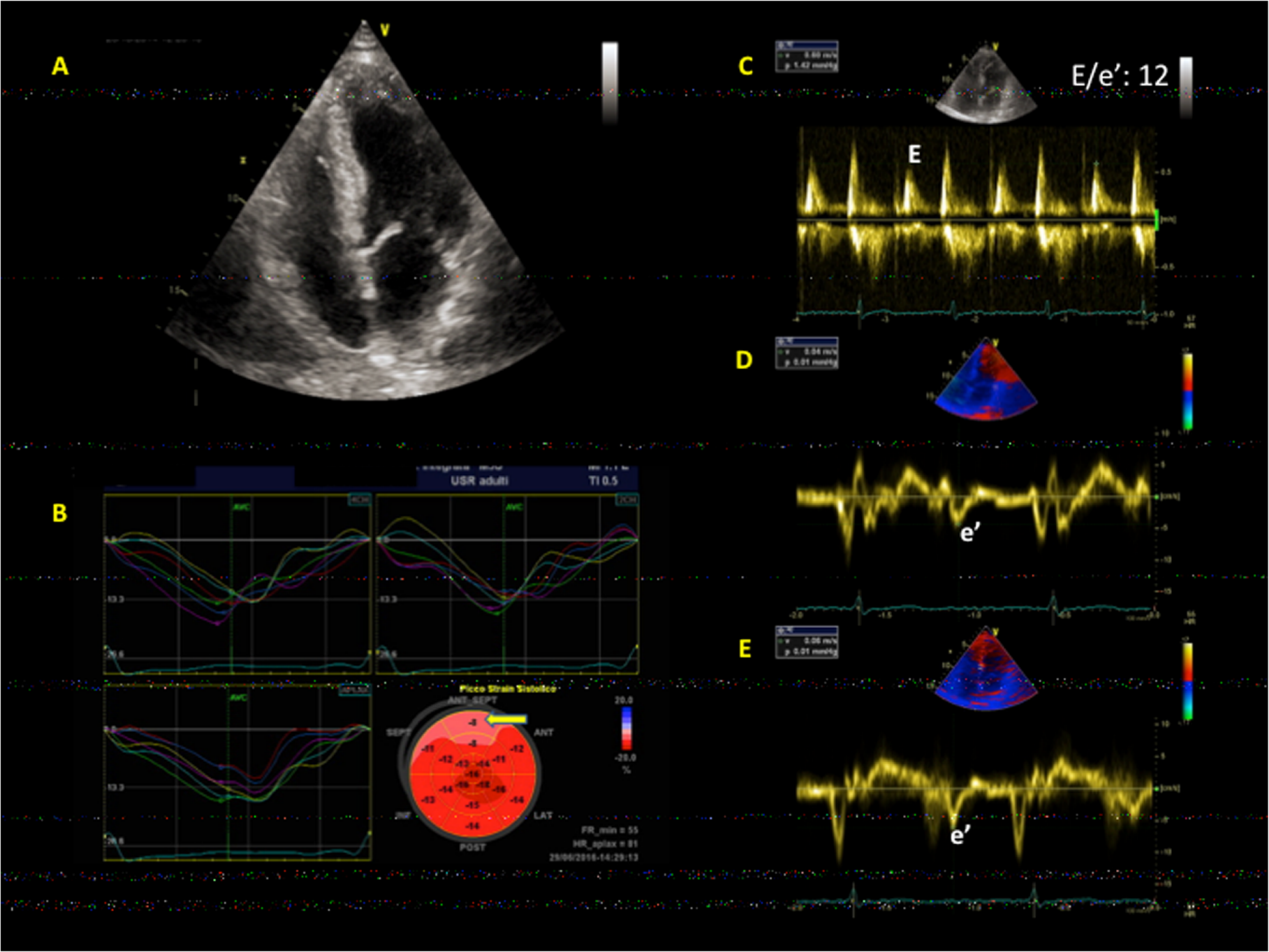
Left ventricular systolic and diastolic dysfunction in OSA patients. Two-dimensional echocardiography (a: apical four chamber view) showing mild impairment of resting LV regional and global strain (mainly in the septal region, see arrow) (b) and significant diastolic dysfunction assessed by transmitral flow pattern (c) and both lateral (d) and septal (e) pulsed Doppler tissue imaging
